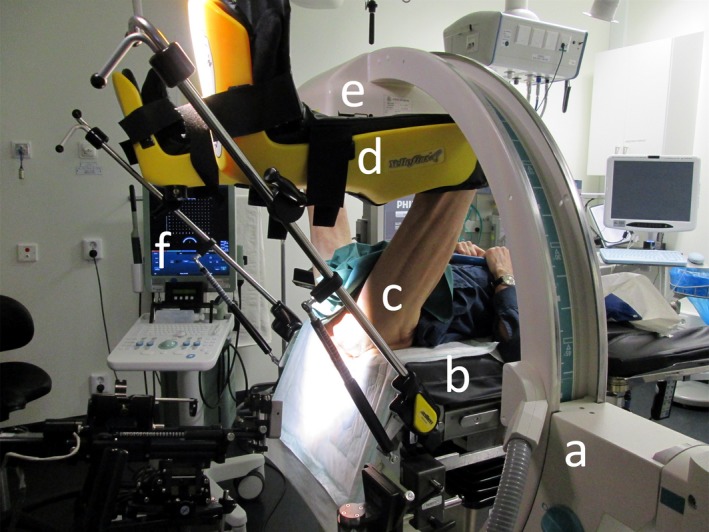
C‐arm X‐ray patient positioning. The patient (c) was placed in a lithotomy position during the transperineal ultrasound (f) guided implantation of the GFM. The legs were fixated using a leg support (d). The C‐Arm X‐ray system (a), with the X‐ray detector (e), was placed in a zero degree angle with respect to the patient table (b) to acquire a posterior‐anterior X‐ray for a successful GFM implantation verification.
